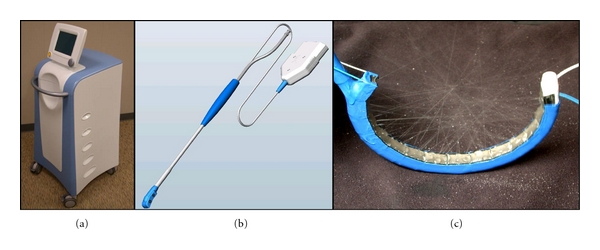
Modern high-intensity focused ultrasound (HIFU) ablation device, Epicor^TM^ System Console (a), Epicor^TM^ UltraWand LP (b) and medical UltraCinch LP ablation device (c). LP: low profile.
